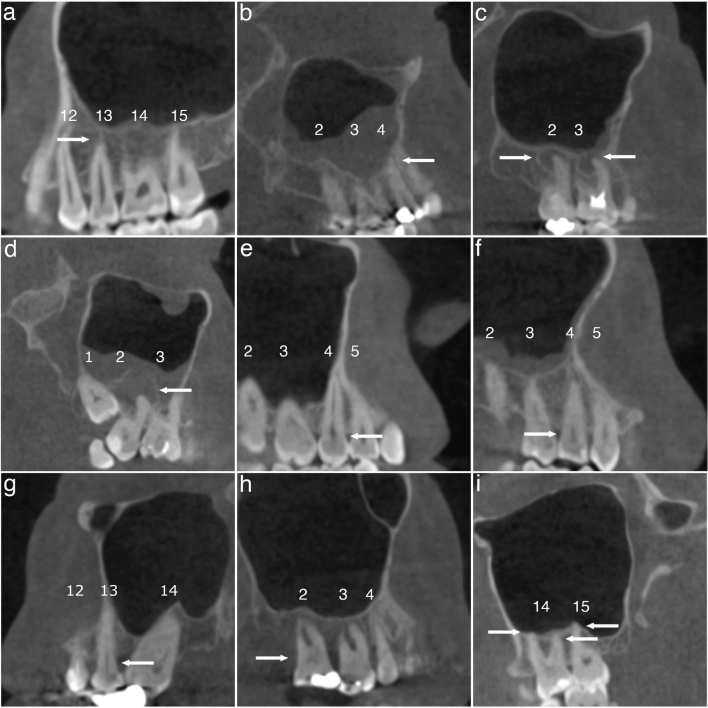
Representative CBCT scans of dental findings. Categories of periapical lesions: (a) normal; (b) widening of the radiolucent periodontal ligament space and minor changes of radiopaque lamina dura; (c) periodontitis with well-defined and corticated radiolucent area; (d) severe periodontitis with exacerbating structures. Categories of periodontal bone loss; (e) normal (2 mm or less); (f) mild (2.1 – 3 mm); (g) moderate (3.1 – 5 mm); (h) severe (more than 5 mm). (i) At least one of the tooth roots in contact with the maxillary sinus floor. Teeth are numbered according to World Dental Federation notation (FDI) and each category/finding is indicated by arrows.
